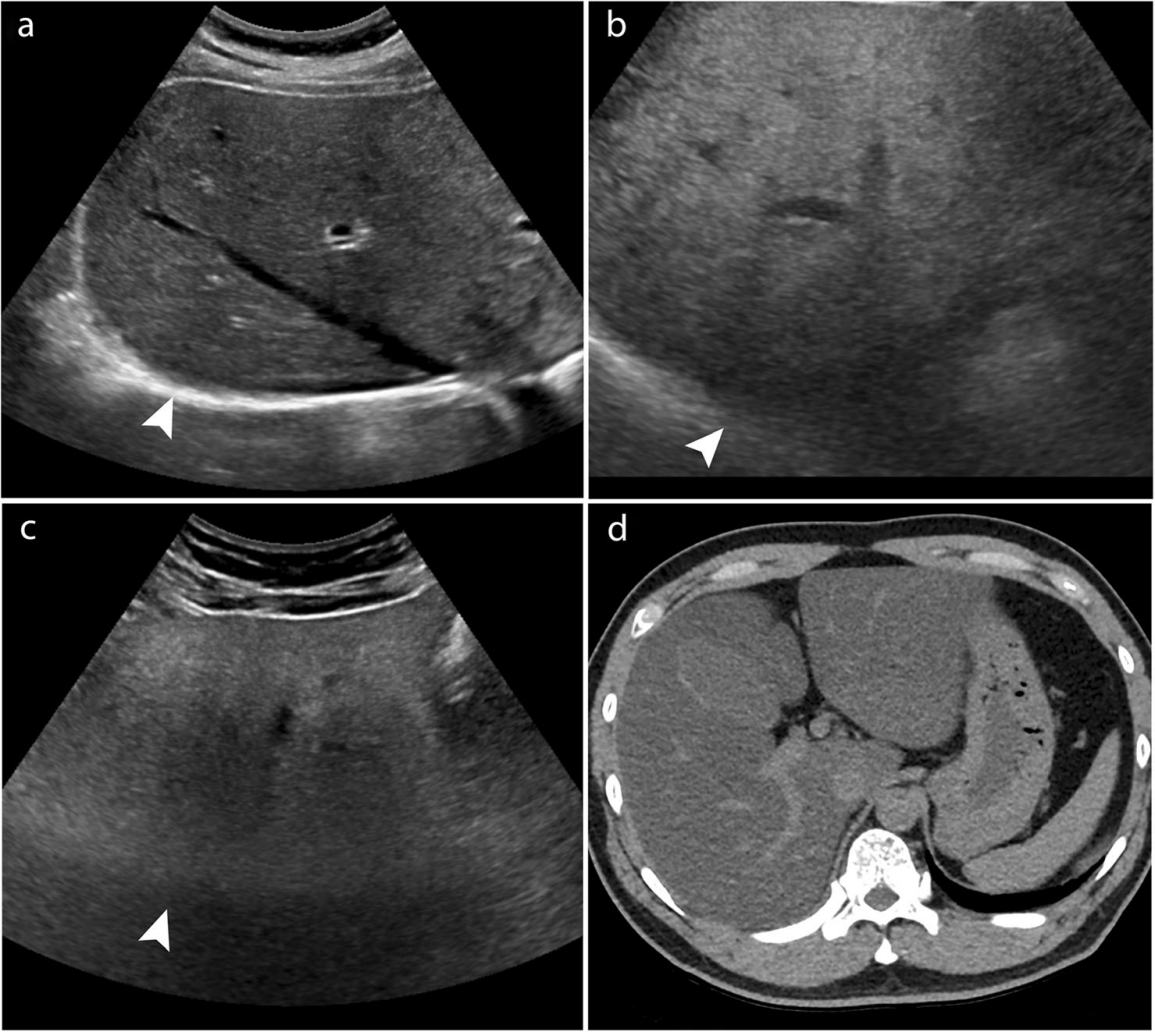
Liver Imaging Reporting and Data System ultrasound visualisation scores. a Example of ultrasound visualisation score A: no or minimal limitations with complete visualisation of the diaphragm (arrowhead). b Example of ultrasound visualisation score B: moderate limitations. Shadowing and attenuation from heterogeneous liver parenchyma may obscure small masses and less than 50% visualisation of the liver (arrowhead). c Example of ultrasound visualisation score C: severe limitations. Marked attenuation in a patient with severe fatty liver leads to poor visualisation of the majority of the liver and diaphragm (arrowhead), with corresponding computed tomography image shown in (d)
